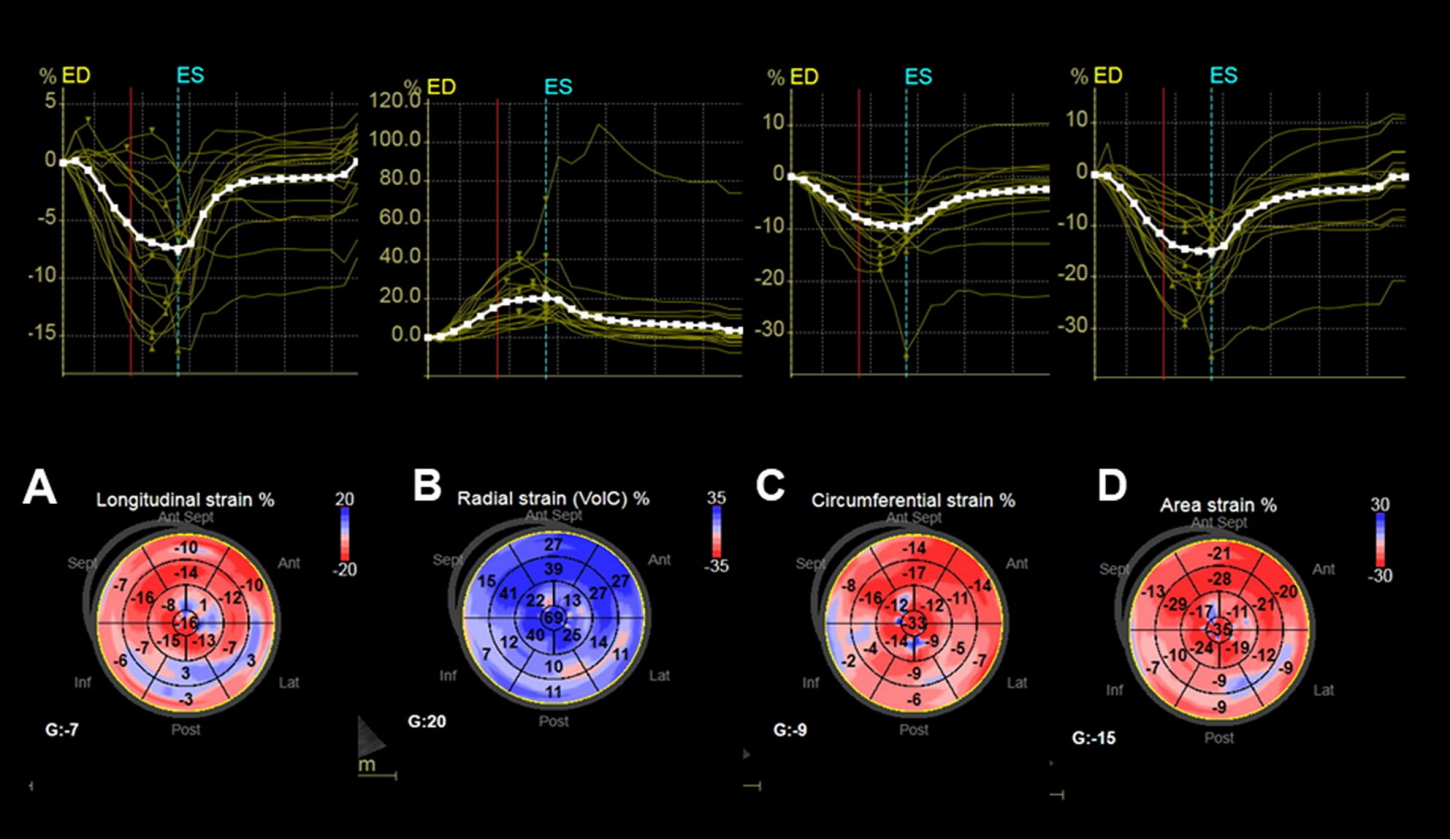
3D STE images from a patient with CD cardiomyopathy and severe LV dysfunction. (A) 3D LV global longitudinal strain = − 7%. (B) 3D LV global radial strain = 20%. (C) 3D LV global circumferential strain = − 9%. (D) 3D LV area strain = − 15%. 3D STE parameters are reduced diffusely. *3D STE* Three-dimensional speckle tracking echocardiography, *CD* Chagas disease, *LV* left ventricular.
